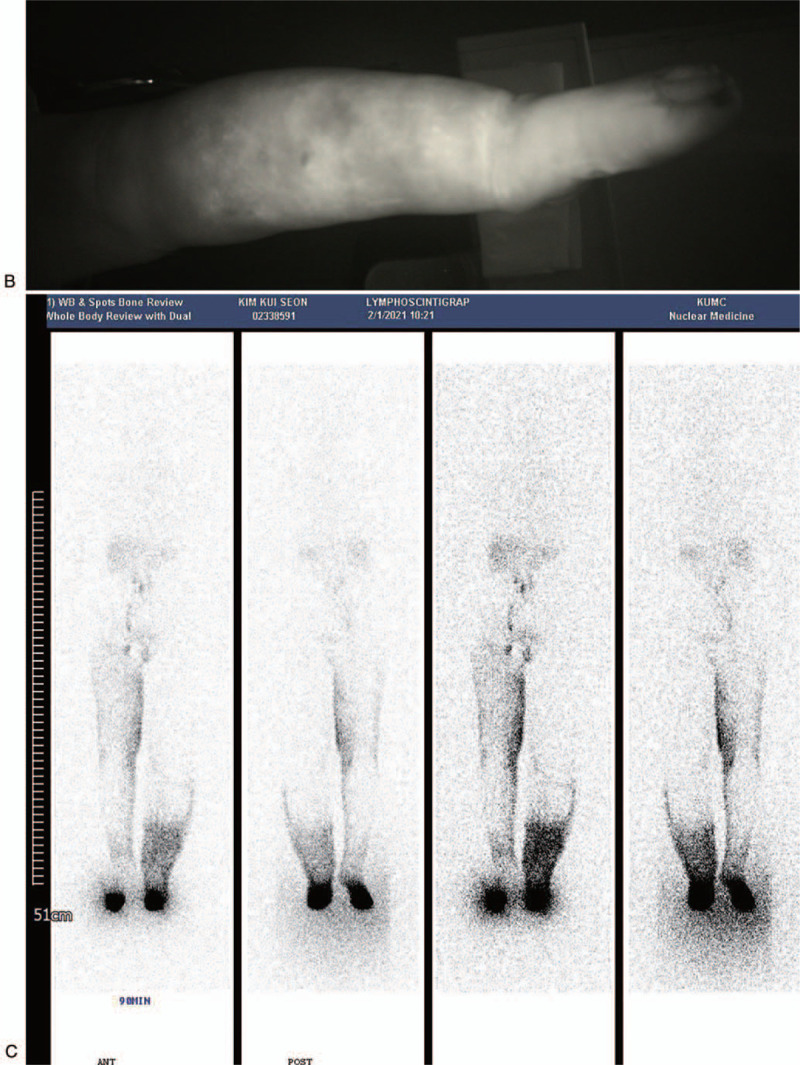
A patient with vasculopathy and severe lower extremity lymphedema. (A) Computed tomography angiography. (B) Indocyanine green lymphography. (C) Lymphoscintigraphy. (D) Magnetic resonance lymphangiography. This patient had International Society of Lymphology stage 3 lymphedema (end-stage lymphedema) with confluent dermal backflow and no functional lymphatic vessels were visualized in the indocyanine green lymphography.
